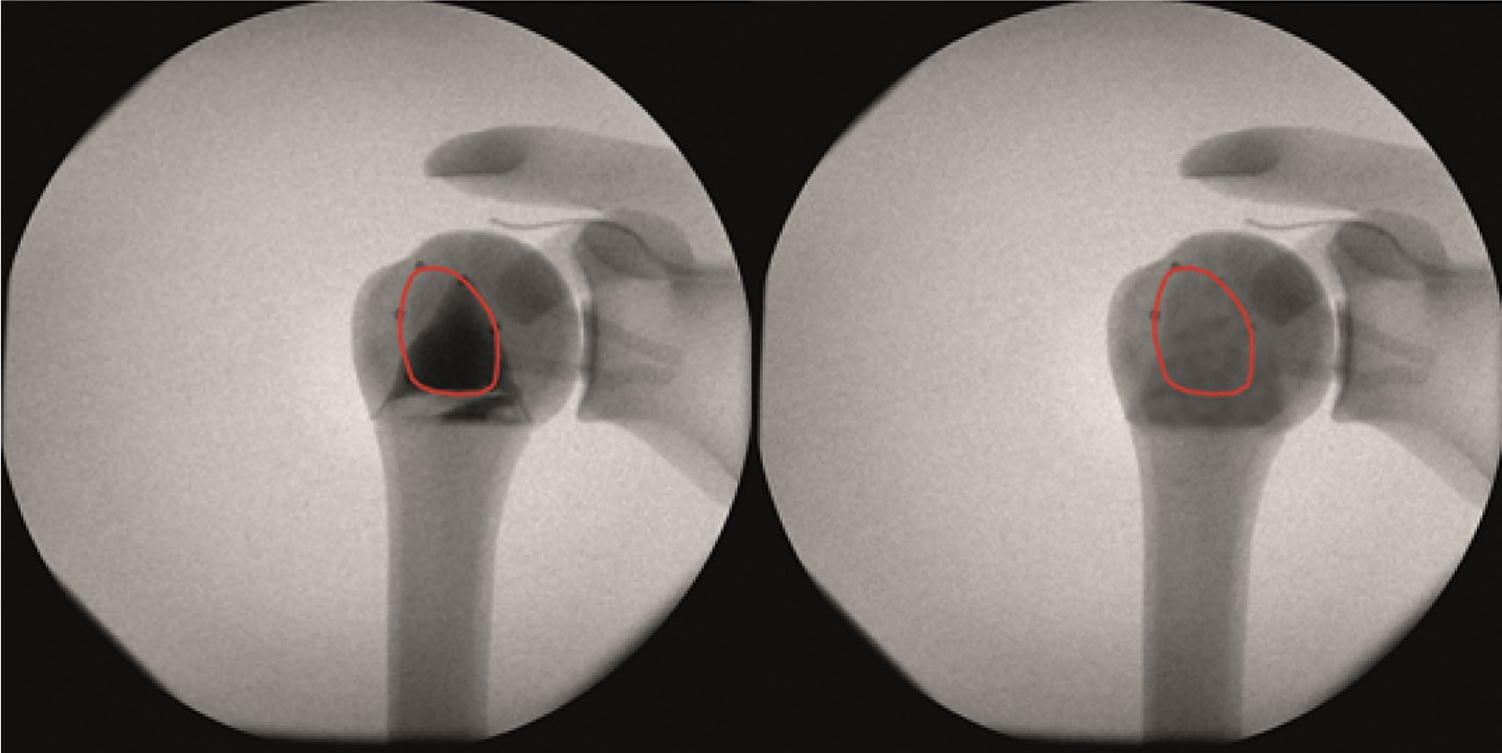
Anteroposterior view radiograph of the saw bone shoulder prepared with the 2-part fracture before (left) and after (right) image editing
